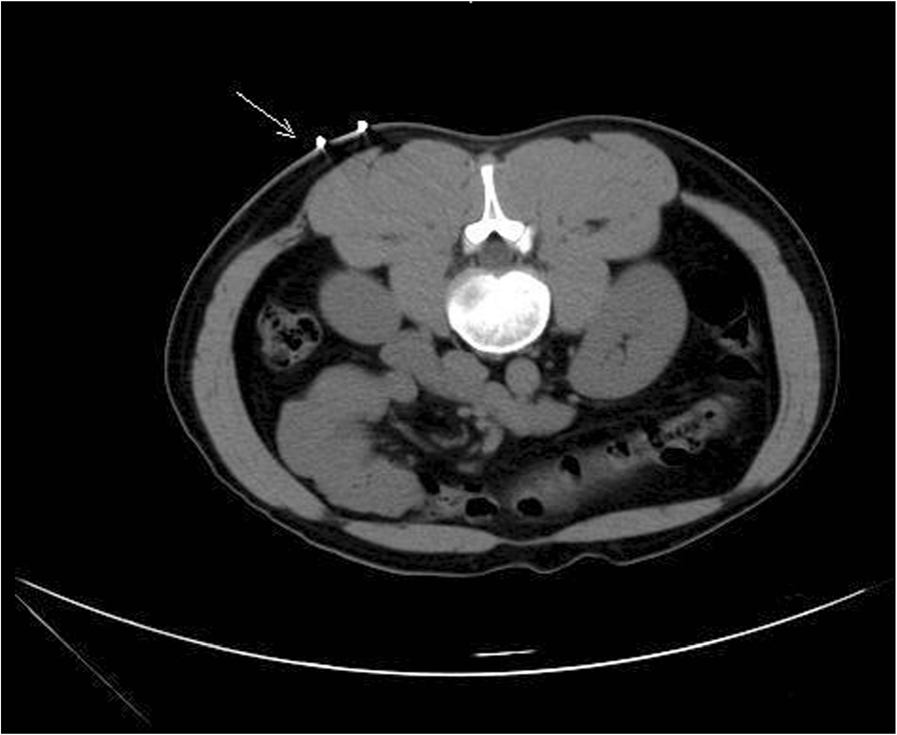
5.0 mm axial CT scan was taken through the lower pole of the kidney to determine the puncture site (Standard-dose scanning).
